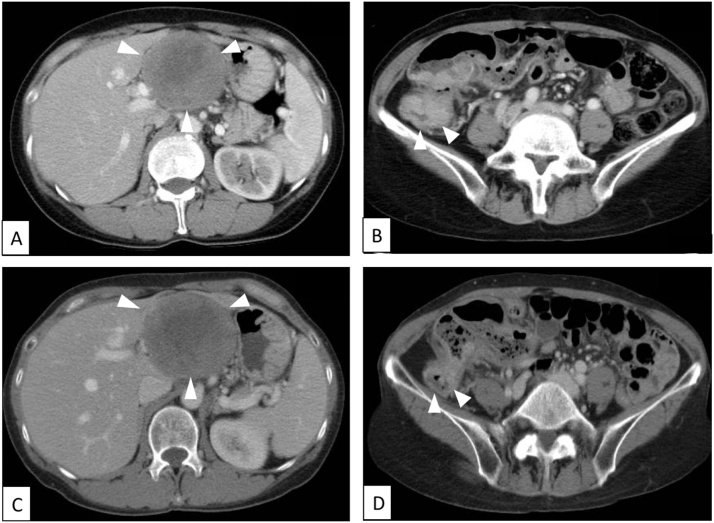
CT images before (A, B) and after (C, D) chemotherapy. A 7.0 × 6.0 cm solid tumor apparently located in the left lobe of liver (A, C) and luminal narrowing with marked wall thickening involving the ascending colon (B, D) are seen. The colon lesion has become smaller with chemotherapy but the size of the liver lesion has not changed significantly.
